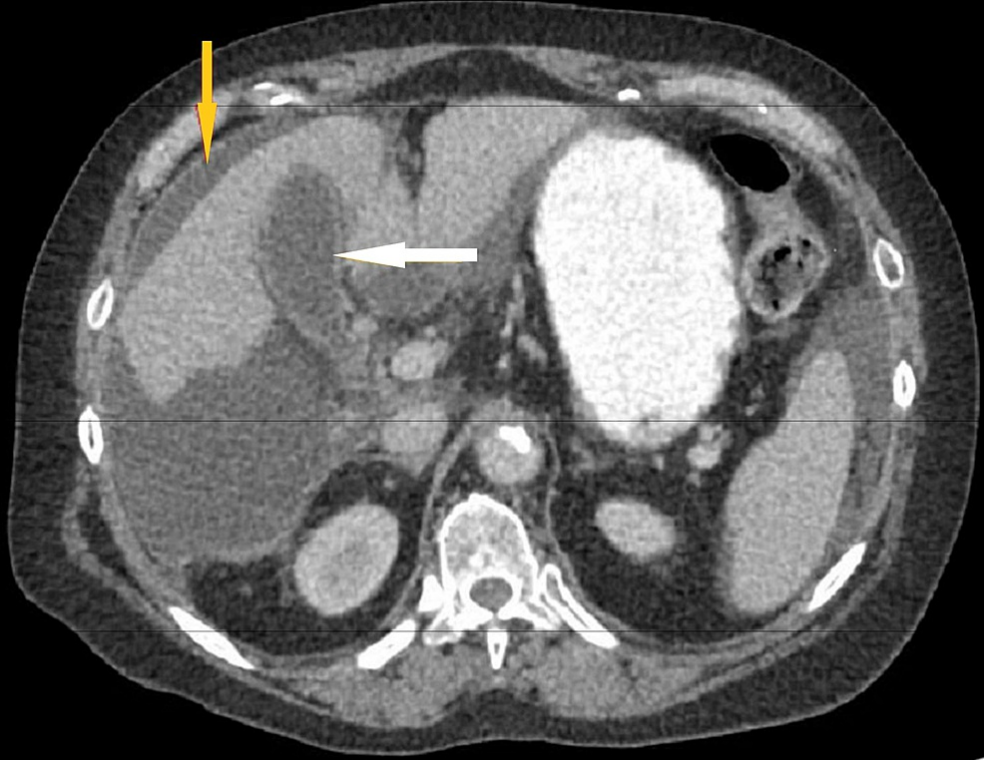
Computed tomography scan of the abdomen and pelvis with contrast. The white arrow shows an intact gallbladder without gallstones or evidence of inflammation. The yellow arrow shows fluid from the extrahepatic biloma, tracking along the anterior and lateral hepatic margins.
